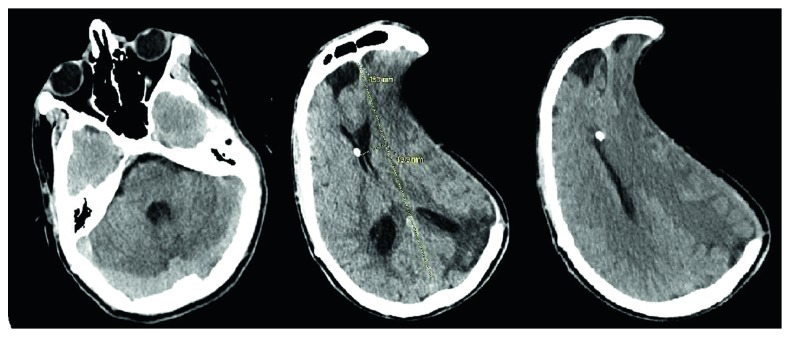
CT head showing left-sided prior craniectomy with significant midline shift.
